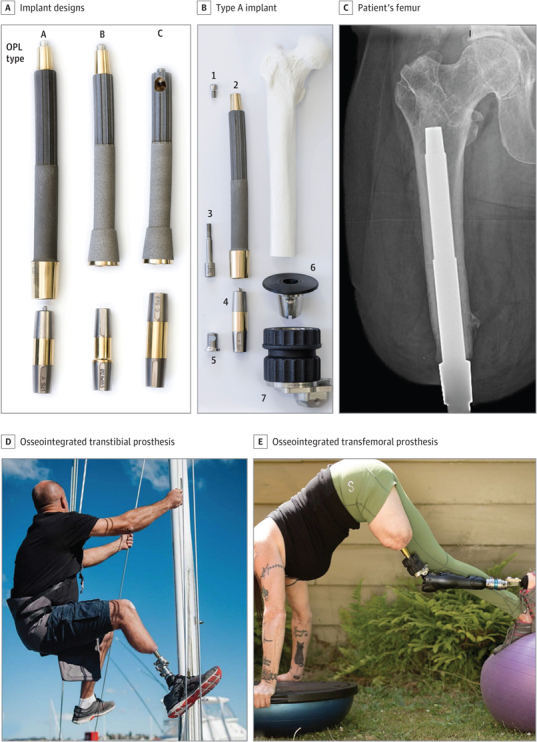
Osseointegration implant and patient examples. (a) Photograph of common osseointegrated prosthetic limb (OPL – Permedica) implant designs. The top portion is made of titanium with a textured intramedullary portion and a smooth transcutaneous portion and is implanted into the patient's residual limb. The dual cone below the implant is a connector to the external prosthetic limb. (b) Exploded view of a type-A implant with the components arranged at approximately the proximal–distal levels in which they would be assembled and implanted in a patient who had undergone a femoral amputation. The numbers in (b) indicate the following: 1 – proximal cap screw, 2 – OPL body, 3 – safety screw, 4 – dual-cone transcutaneous adapter, 5 – prosthesis adapter screw, 6 – proximal connector, and 7 – prosthetic connector. (c) Anterior–posterior radiograph of a TOFA implant in a patient's femur. The textured portion is intramedullary; the smooth portion is transcutaneous. Panels (d) and (e) are examples of activities that a patient with an osseointegrated transtibial ((d) or transfemoral (e)) implant can achieve with a skeletally connected prosthesis. Figure reused with permission from Hoellwarth et al. (2022a).
